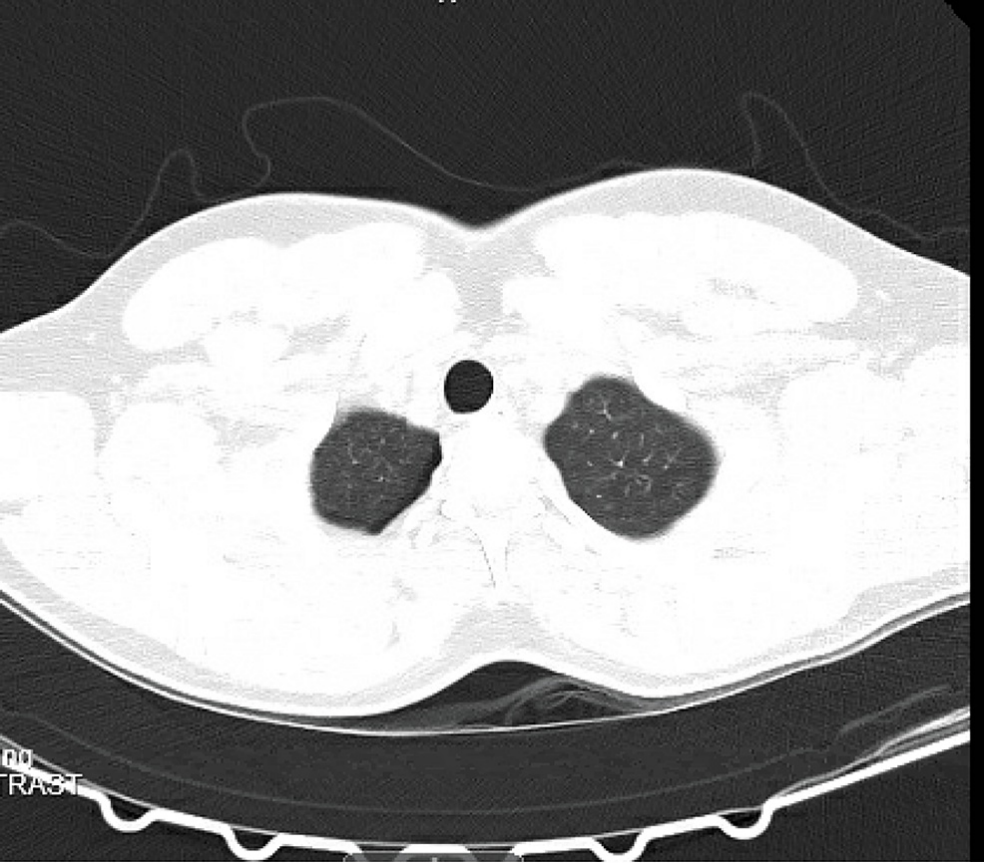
Computed tomography showing small remaining right apical pneumothorax
